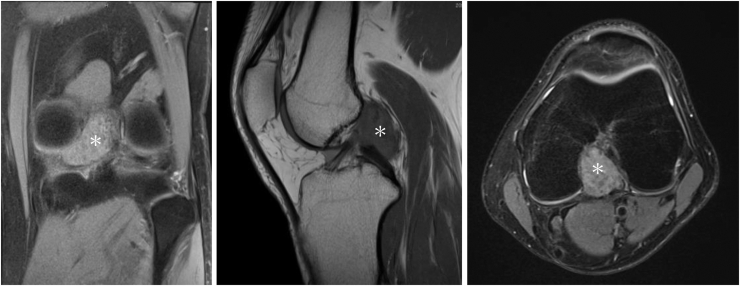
Preoperative magnetic resonance imaging of the left knee showing the localized pigmented villonodular synovitis (localized pigmented villonodular synovitis: white asterisk) in the posterior knee compartment, highlighting the extent and anatomic location of the lesion.
